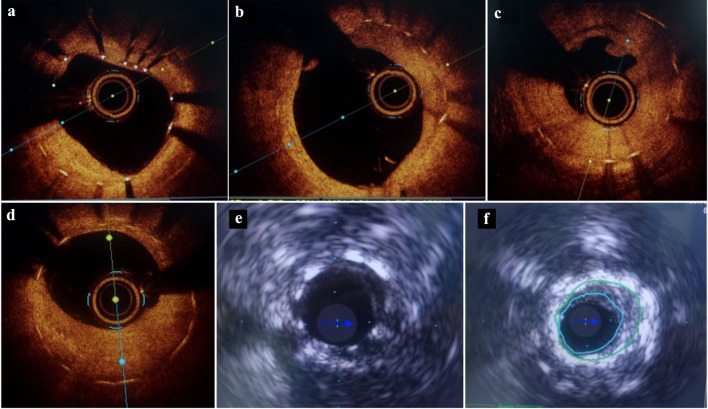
Representative OCT and IVUS images demonstrating mechanisms of ISR. Panels (a)–(d) show optical coherence tomography cross-sectional images of ISR. Panel (a) demonstrates eccentric neointimal hyperplasia within the stented segment causing significant luminal compromise with well-apposed stent struts. Panel (b) shows diffuse, predominantly homogeneous neointimal proliferation resulting in concentric luminal narrowing. Panel (c) demonstrates heterogeneous neointimal tissue with signal attenuation suggestive of complex tissue composition, consistent with neoatherosclerosis. Panel (d) shows concentric neointimal hyperplasia with significant tissue burden leading to luminal narrowing. Panels (e) and (f) show intravascular ultrasound images. Panel (e) demonstrates significant neointimal tissue proliferation within the stented segment without an obvious mechanical abnormality. Panel (f) demonstrates stent underexpansion with reduced minimal stent area and persistent luminal compromise, consistent with mechanically driven ISR. ISR: in-stent restenosis; IVUS: intravascular ultrasound; OCT: optical coherence tomography.
